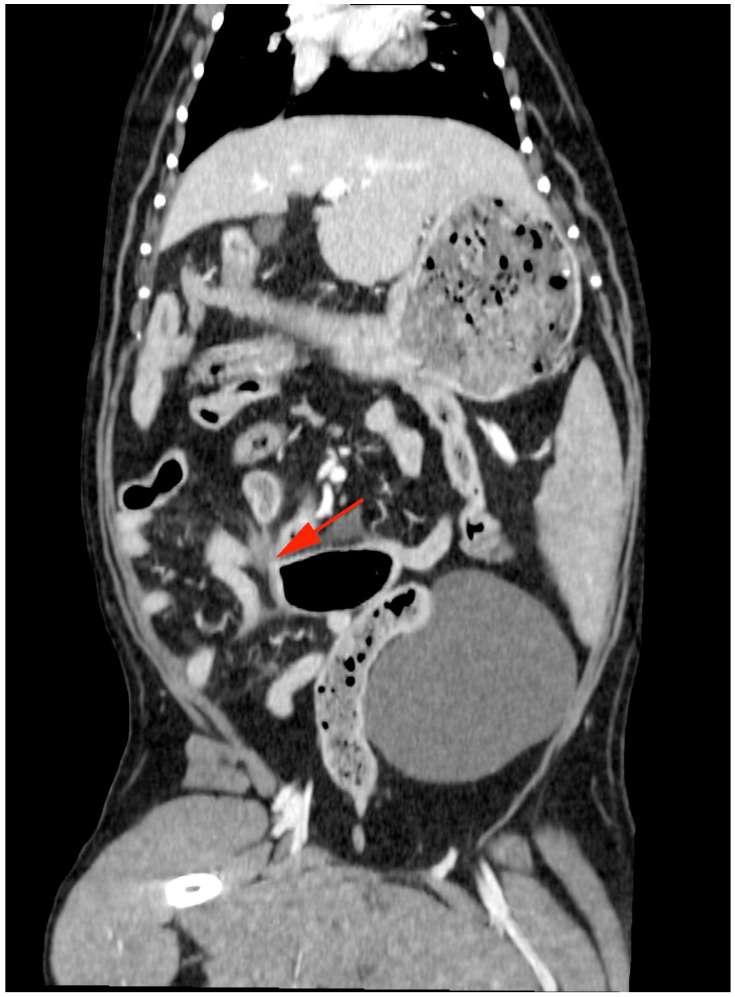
Abdominal CT in the transverse plane displaying the caudal segment of dilated jejunum containing gas and tapering abruptly (red arrow).
